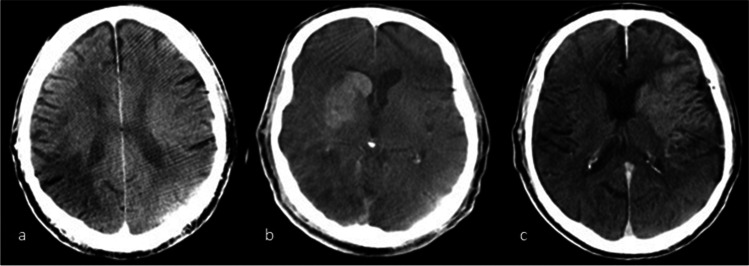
Examples of FDCT with different patterns of demarcation. (a) Purely hypoattenuated pattern (ASPECTS 9). (b) Purely hyperattenuated pattern (ASPECTS 7)*.* (c) Mixed pattern (ASPECTS 2): hyperattenuation in Ncl. caudatus, putamen, internal capsule, insular cortex, M1/2, and M5 (representative slice not shown) hypoattenuation in M3 (nomenclature after Barber et. al. [11])
